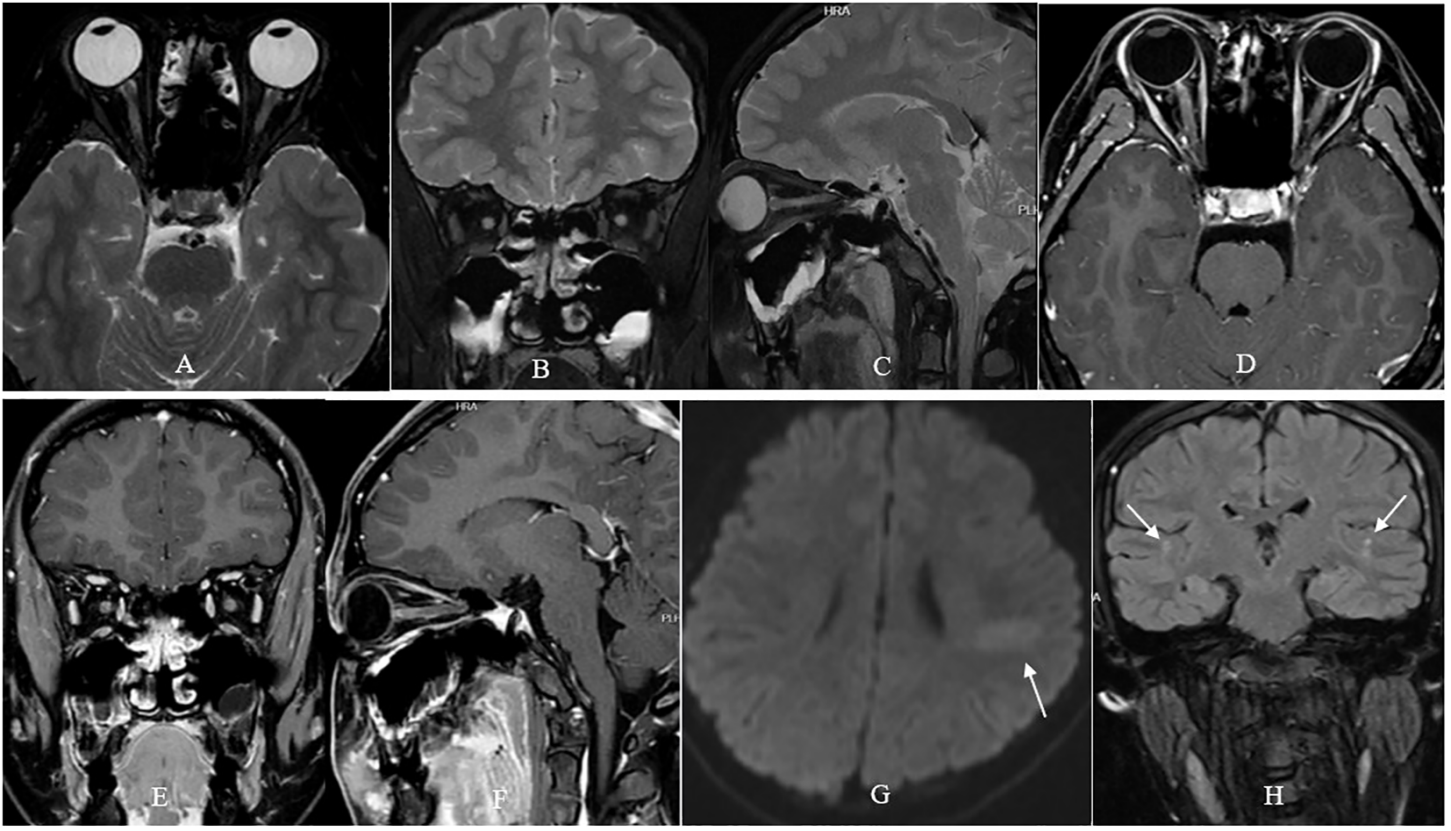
Orbital MRI examination of the patient. T2- fat suppression sequence showed long-segment hyperintensity and enlargement of the intraorbital optic nerve in both eyes (A–C). Contrast-enhanced T1- fat suppression sequence showed long-segment contrast-enhancement of the intraorbital optic nerve in both eyes (D–F). Fluid attenuated inversion recovery sequence showed an isolated finger-like slightly hyperintense lesion vertical to left lateral ventricle [arrow, (G)], and several spotty hyperintense lesions in bilateral parietal lobes [arrow, (H)].
